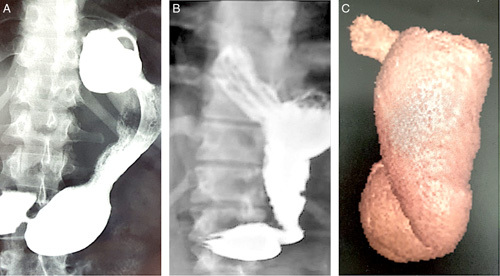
After barium swallow contrast study (A, B) and computed tomography scan volumetric (C). The gastric dilation was classified as primary localized (A, B) or secondary global dilation (C).
